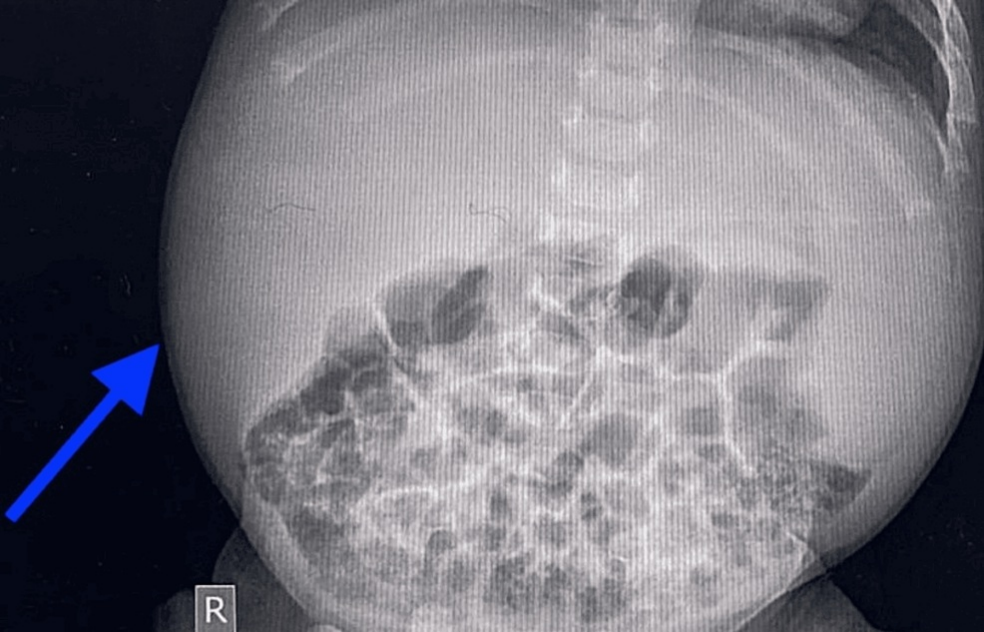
Abdominal X-ray scan of a two-month-old baby girl presented with abdominal destination, hepatosplenomegaly, and diagnosed with congenital syphilis.
